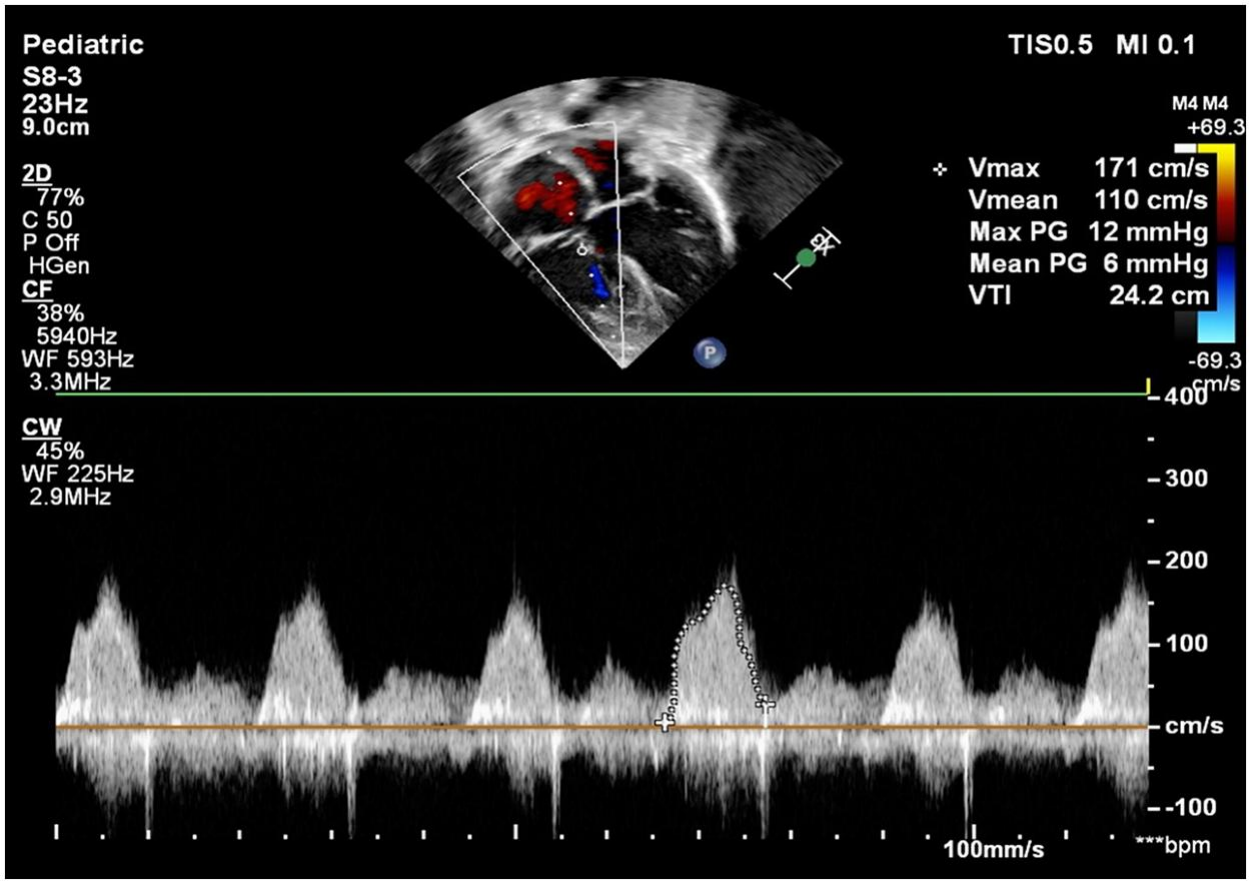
Continuous wave doppler at tricuspid inflow flow showing tricuspid valve stenosis mean gradient of 6 mmHg across tricuspid valve.
